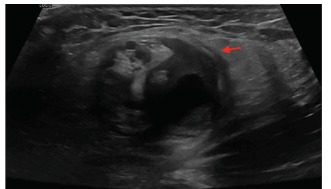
Transverse view of formal ultrasound of the left inguinal canal displaying prominent heterogeneous structures (arrow).
